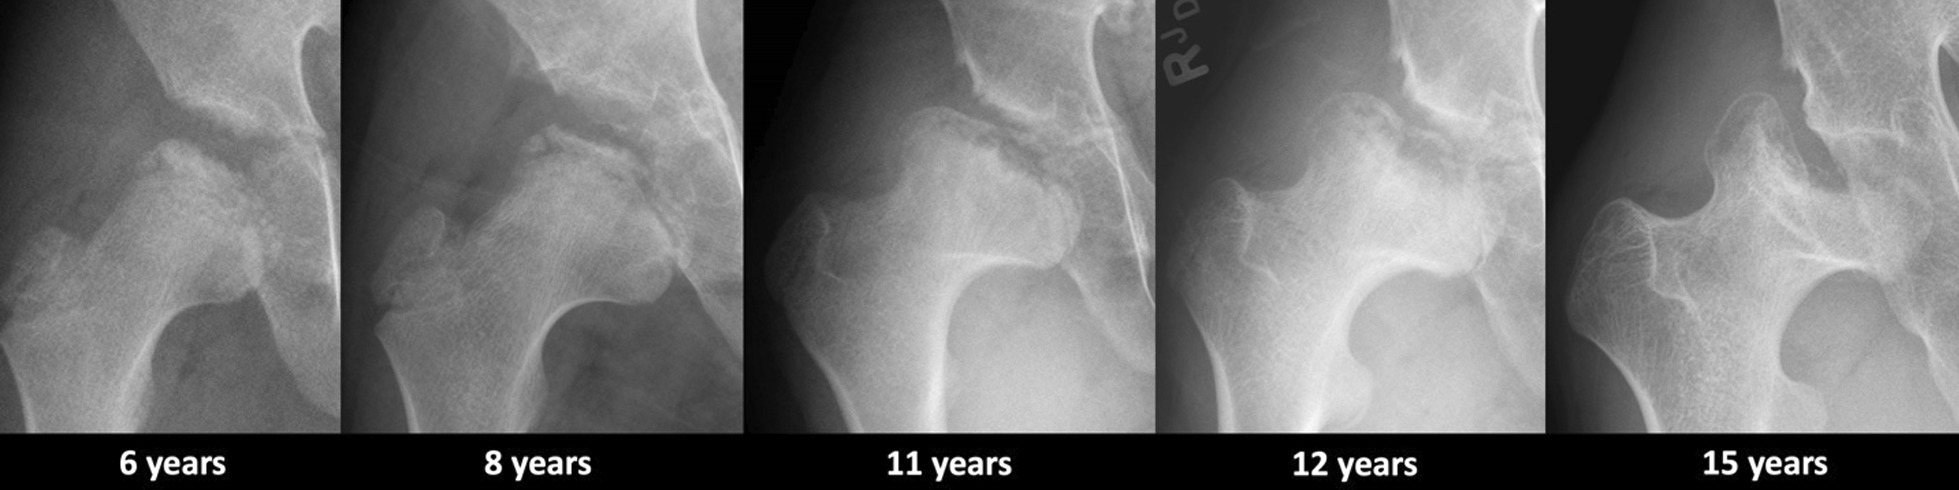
Representative serial radiographs of the proximal right femur of Patient 1 over time show progressive flattening of the epiphysis, concomitant widening of the proximal metaphyses, and increased irregularity of the acetabula resulting in compensatory pseudarthrosis-like formation
